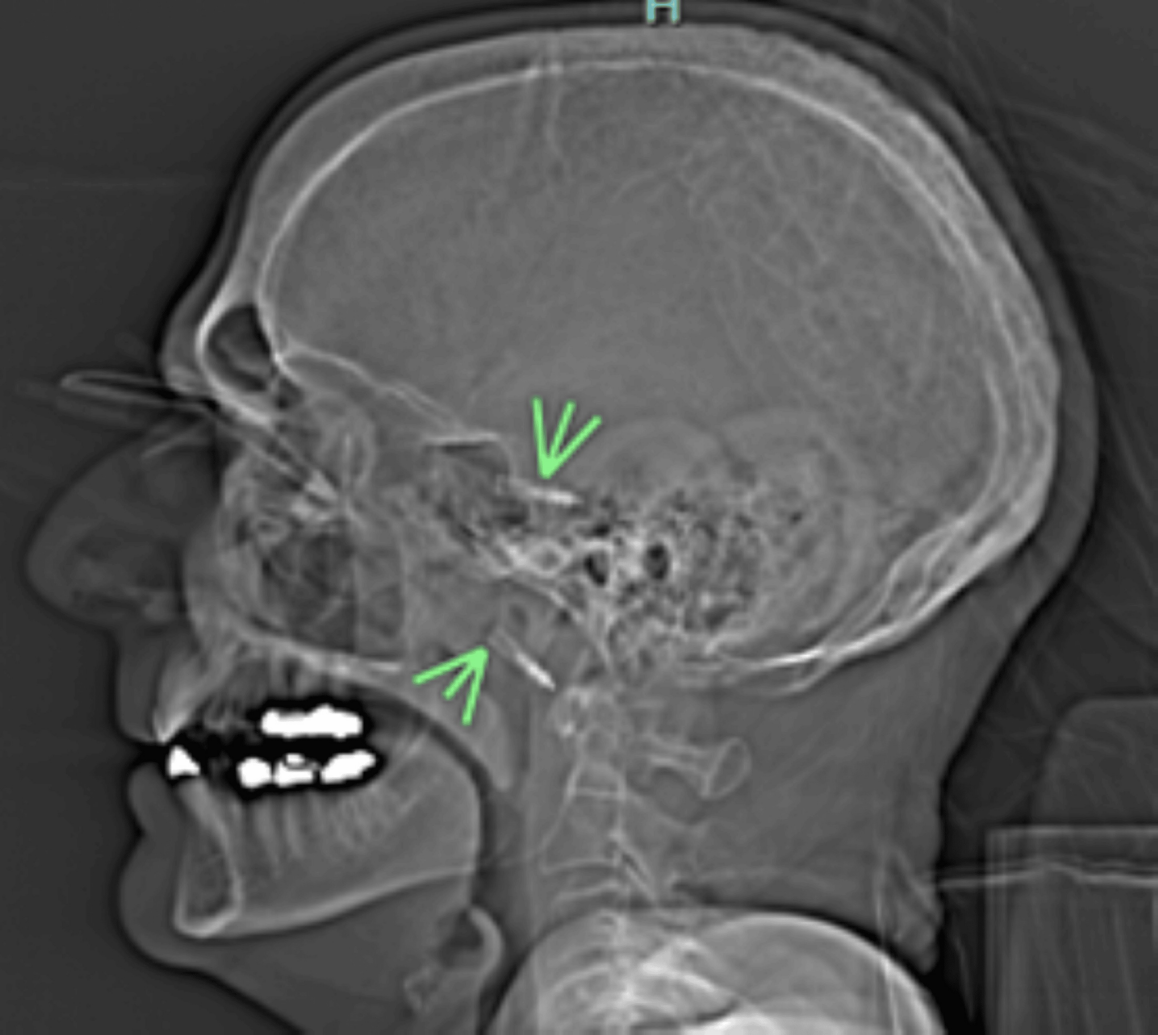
Sagittal computed tomography image showing bilateral orbital penetration by ballpoint pens with intracranial extension.
